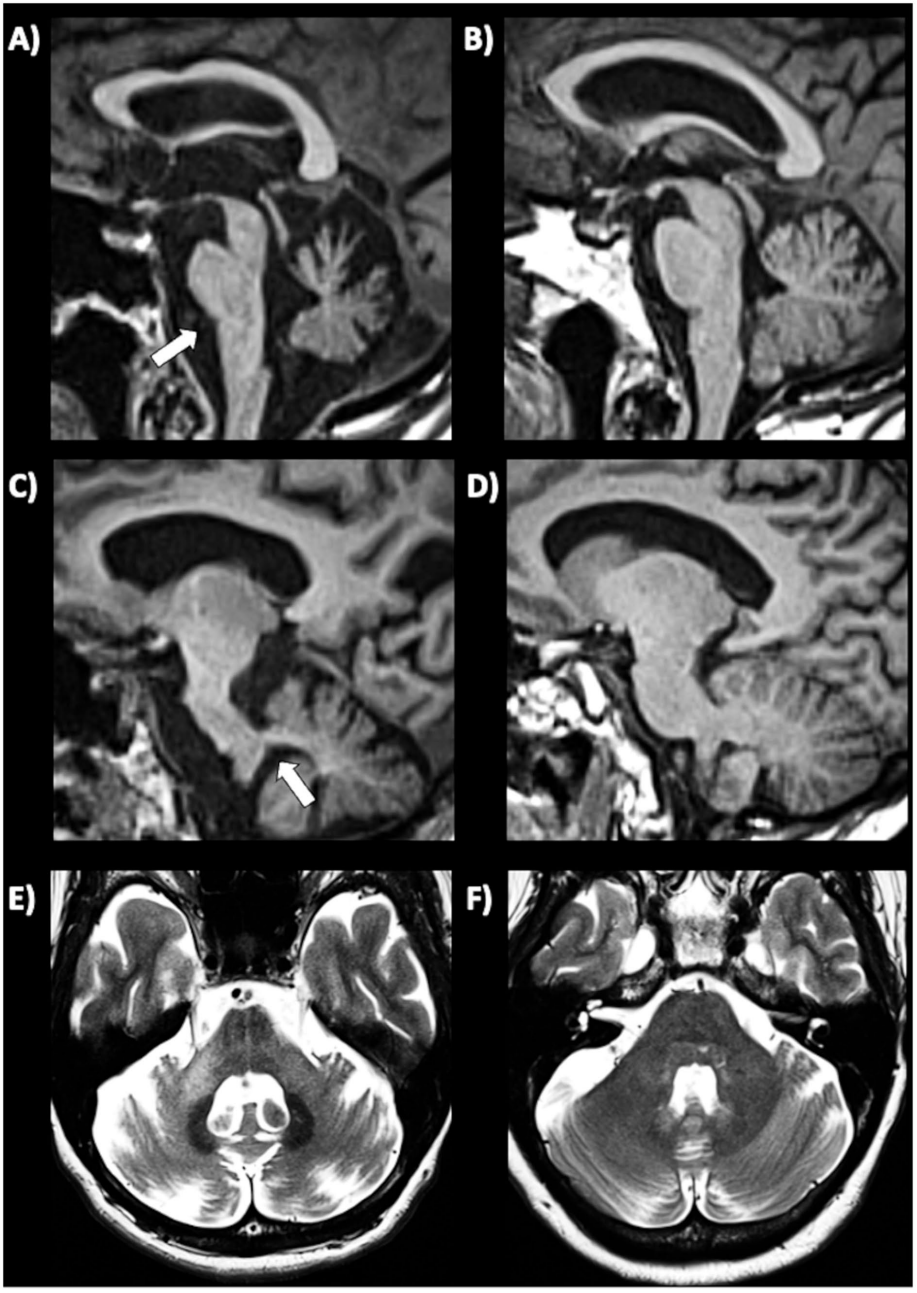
Brain axial 3T-MR images showing infratentorial atrophy in multiple system atrophy. Midsagittal and parasagittal T1-weighted MR images, respectively, show pons atrophy (A) and middle cerebellar peduncle atrophy (C) (highlighted by the white arrows) in a patient with MSA-C, and the normal pons and middle cerebellar peduncle appearance in a patient with PD (B–D). A T2-weighted axial MR image (E) show a cruciform hyperintensity in the pons (“hot cross bun” sign) in an MSA-C patient, and absence of this sign in a PD patient (F). MSA-C, multiple system atrophy—cerebellar type; PD, Parkinson’s disease.
